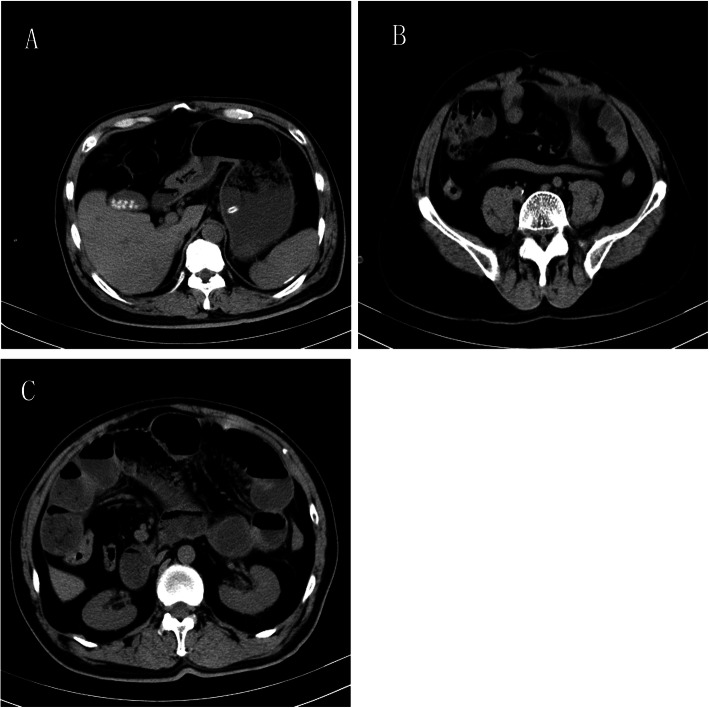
Abdominal CT at admission. a: Gallstones. b: Congenital intestinal malrotation. c: Small intestine obstruction with envelope wrap, fluid density around the liver can be seen, multiple gas in the intestine can be seen, accompanied by gas and liquid level
